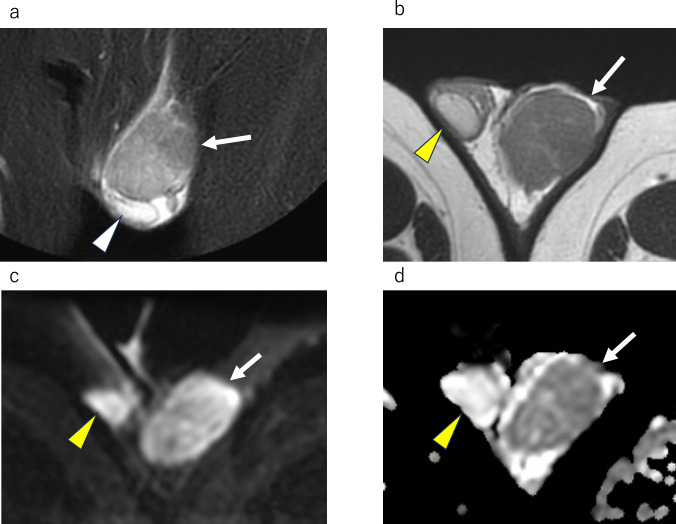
Leiomyosarcoma in a 60-year-old male with a left scrotal mass enlargement 3 months ago. a Coronal fat-suppressed T2-weighted MR image shows mild hypointense mass (white arrow) superior to the left testis (white arrowhead). There is no invasion of the testis. b Axial T2-weighted MR image shows mild hypointense mass with lobulated shape (white arrow). The yellow arrowhead indicates the normal right testis. c Axial diffusion weighted MR image shows a hyperintense mass (white arrow) comparable to that of the normal right testis (yellow arrowhead). d Apparent diffusion coefficient (ADC) images show that the mass (white arrow) has a low ADC value (0.874 × 10^−3^mm^2^/sec) compared to the normal right testis (yellow arrowhead)
